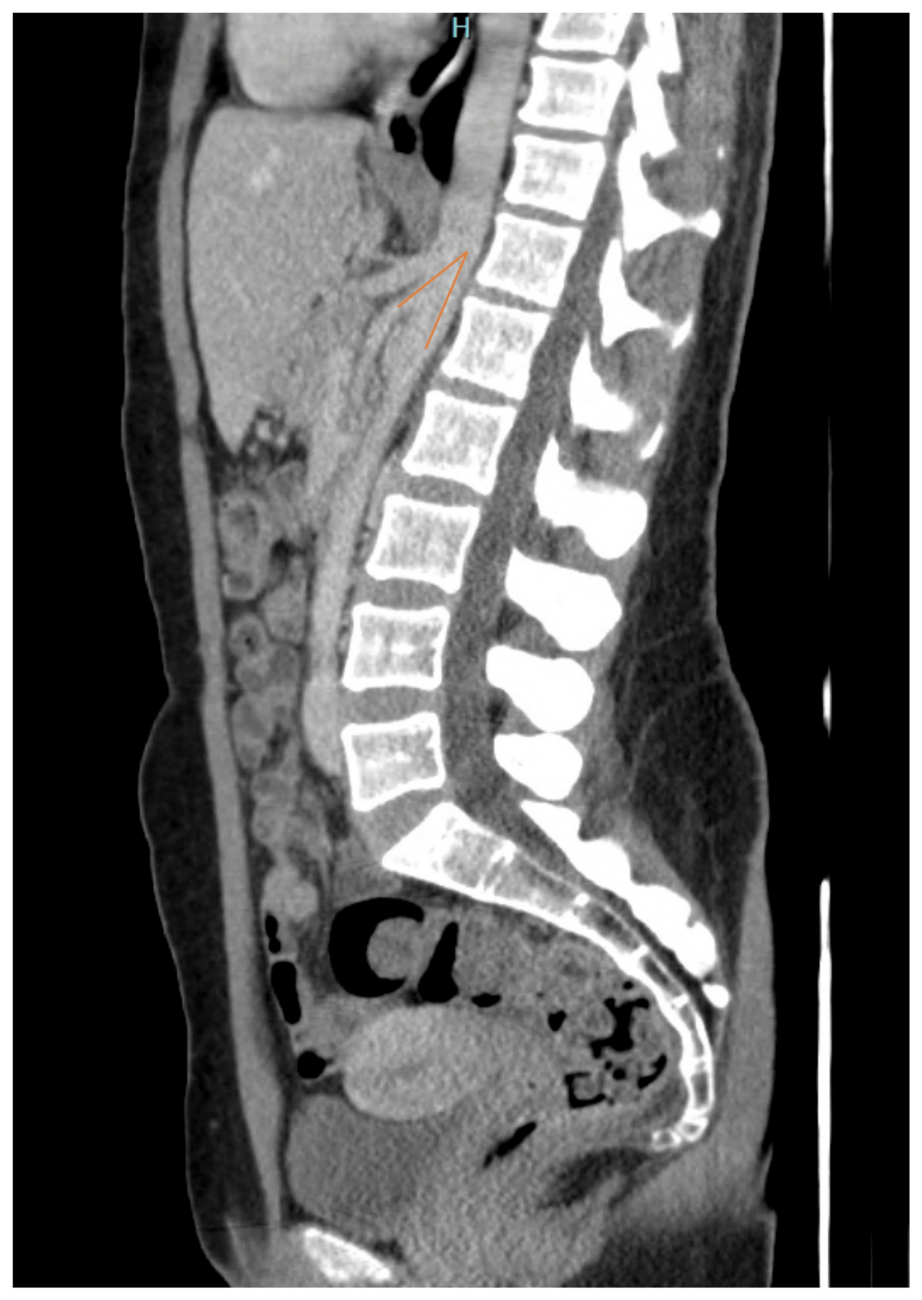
A sagittal view of the CT scan shows the origin of the SMA (left arrow) and the aorta (right arrow), with the compressed LRV in the narrowed space. CT, computed tomography; SMA, superior mesenteric artery; LRV, left renal vein
